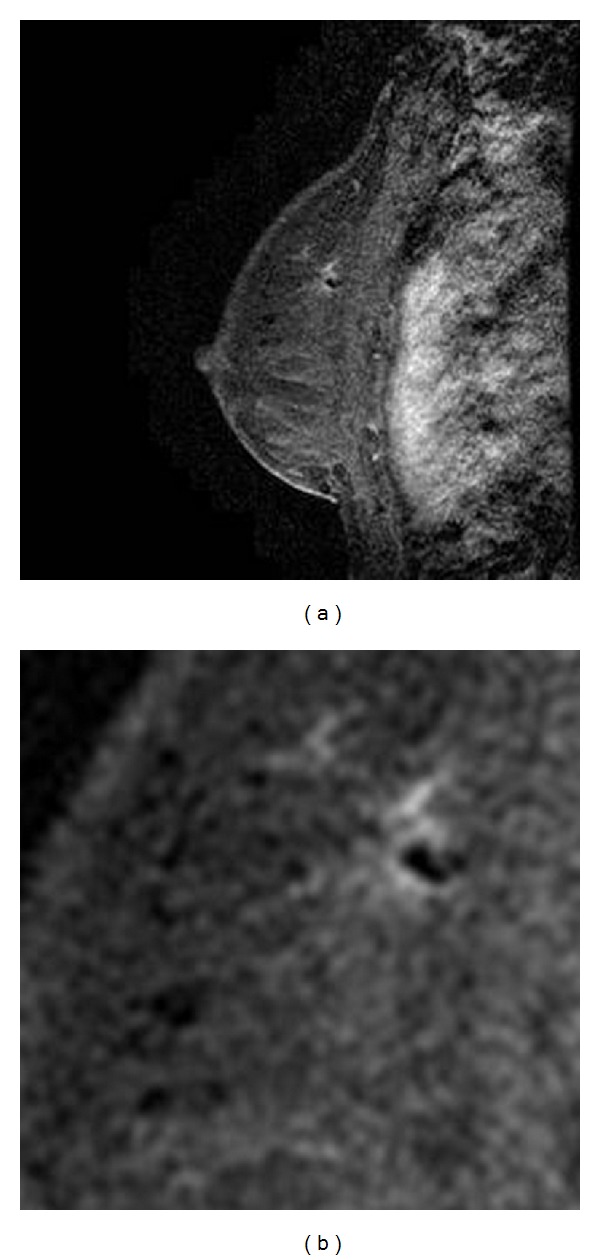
(a) Postclip placement MRI demonstrates a signal void (clip) within the enhancing nodule, confirming that the appropriate lesion was biopsied under ultrasound. (b) Coned down, magnified view of the same area.
